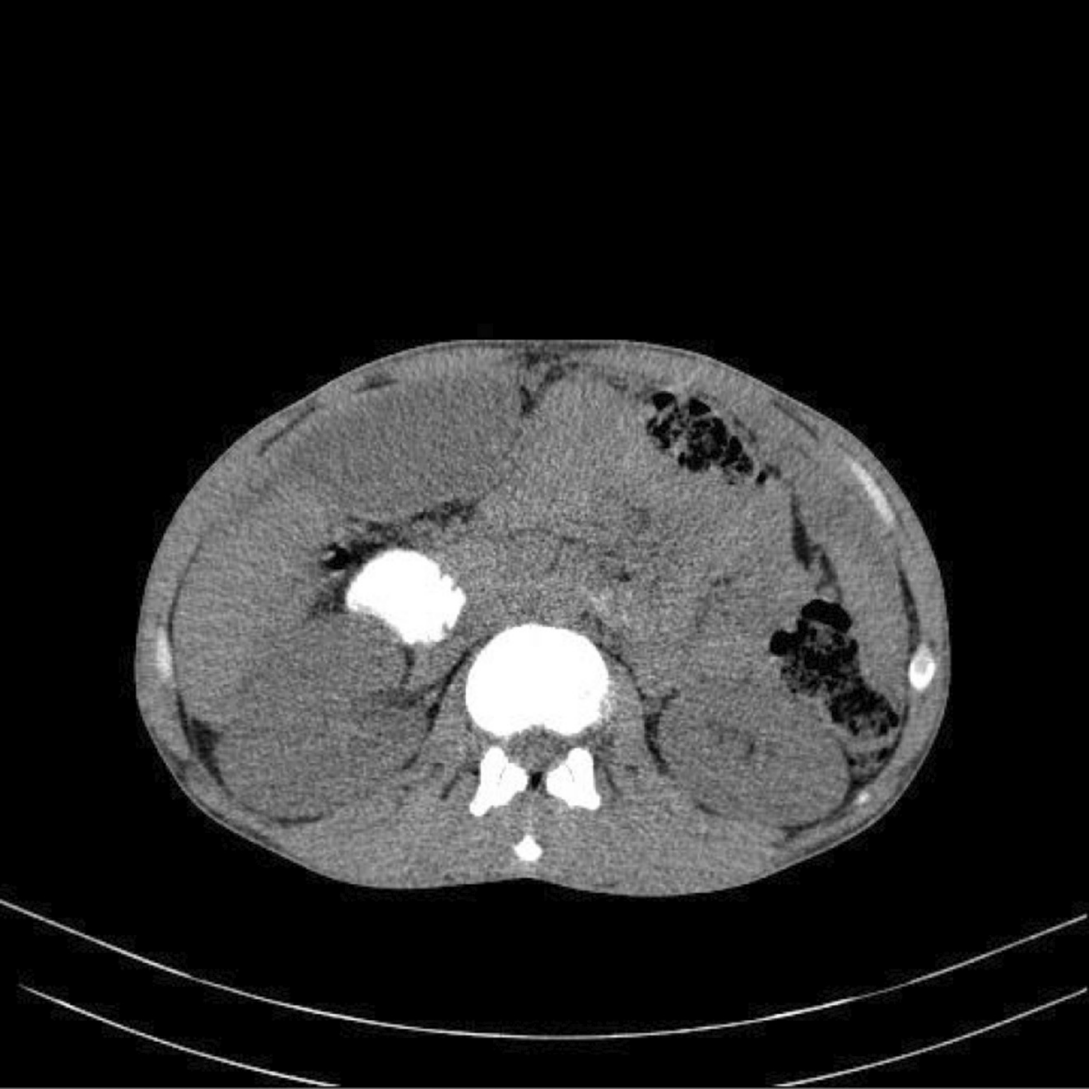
Abdominal CT scan. Increased in the omentum, nodularity, and a subdiaphragmatic abscess (66*148 mm).
